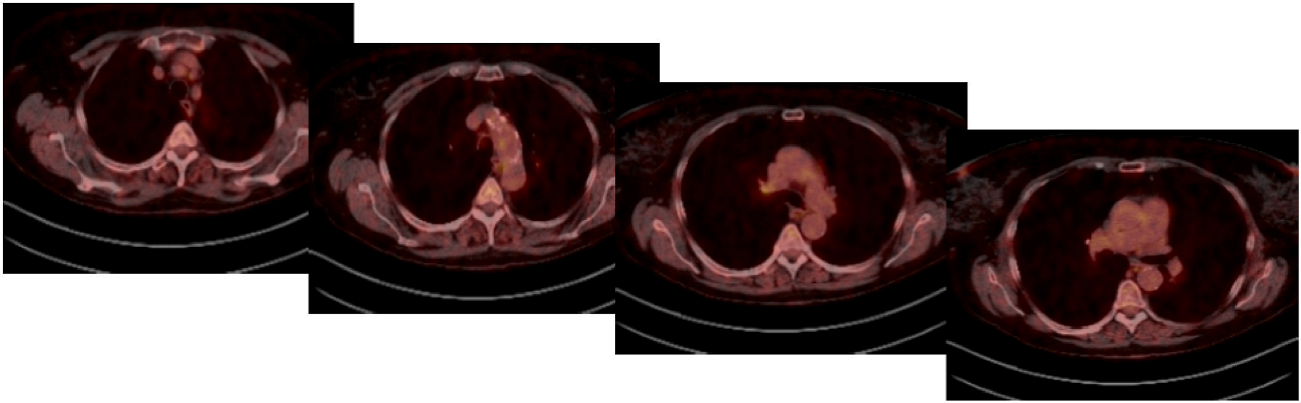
PET-CT-Scan after cycles 3 and 4 of neoadjuvant chemoimmunotherapy: No detectable FDG-uptake in lymph-nodes. Borderline elevated FDG-uptake in the primary lesion.
